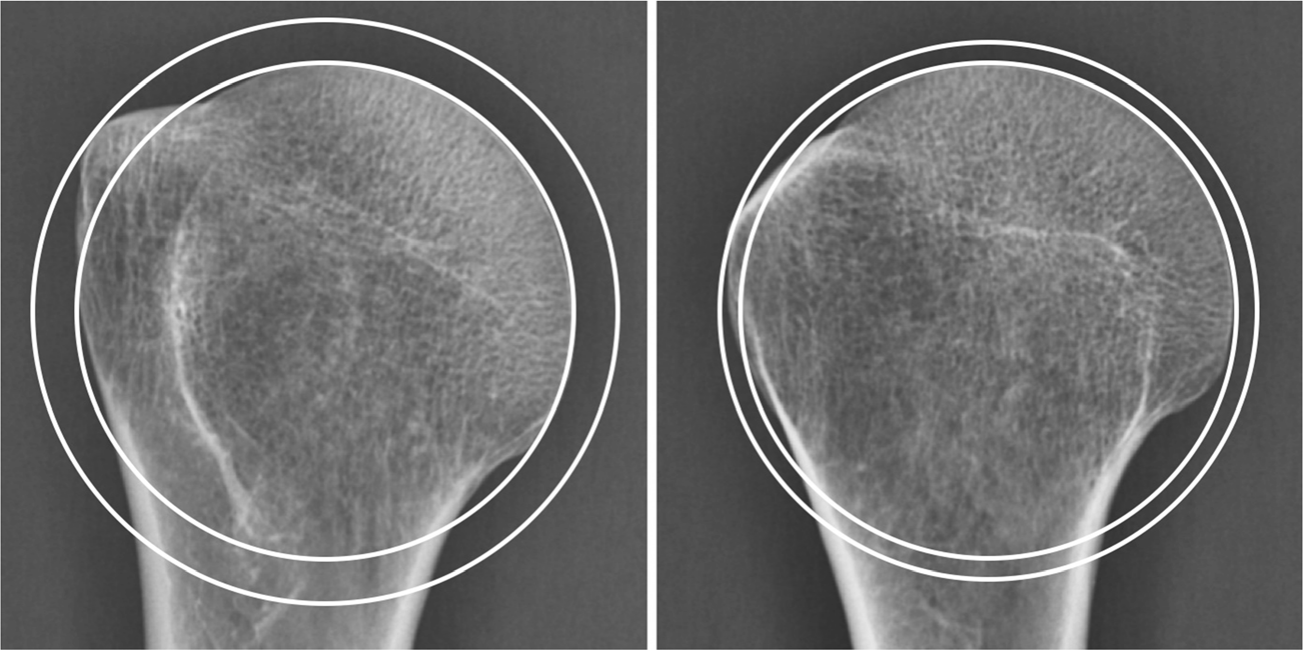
Anteroposterior radiograph of a dry humerus in neutral and internal rotation demonstrating the influence of rotation on the Greater Tuberosity Index. Internal rotation (on the right side) exposes the posteroinferior aspect and hides the superior part of the greater tuberosity, which is relevant for subacromial impingement. The measurements should therefore be made with the arm in neutral rotation
